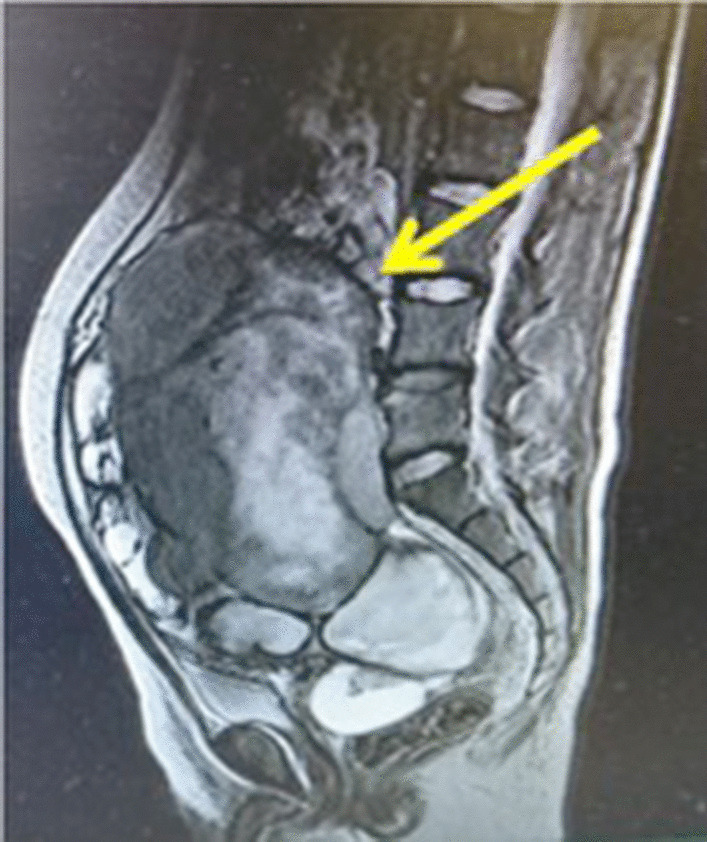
Magnetic resonance imaging findings (T2-weighted image): pelvic tumor with a mixture of solid and cystic components (arrow)
